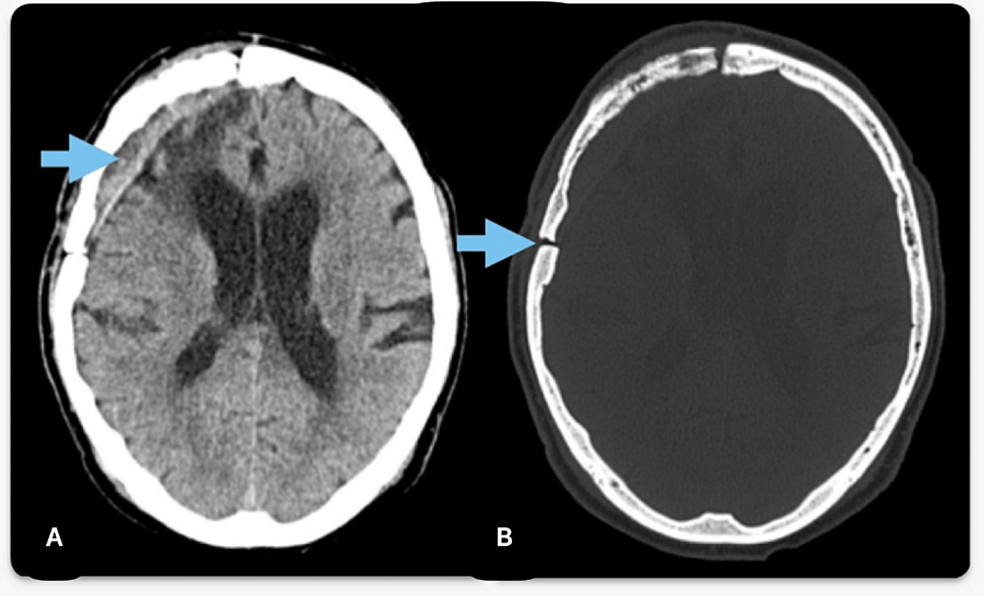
Postoperative CT scan aspect (A) CT tissue window highlights gross tumour resection; (B) CT bone window shows pterional flap on the right side of the skull
